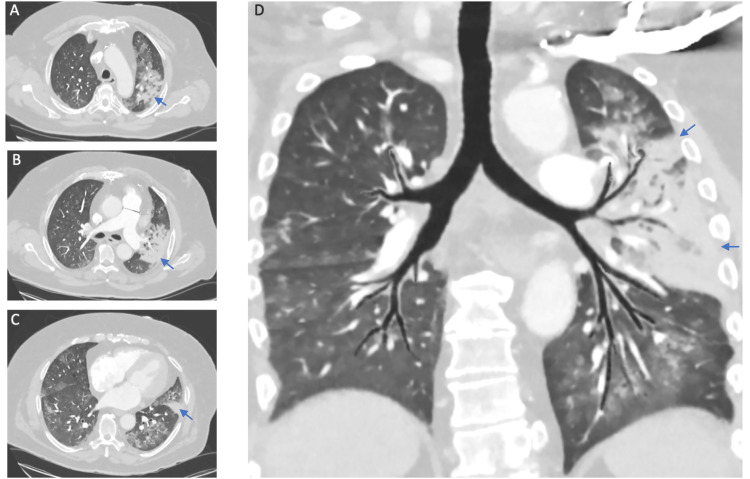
Chest computed tomography angiography Confluent area of consolidation with an air bronchogram in the left upper lobe and diffuse ground glass opacities. A-C: axial views in the upper, medium, and lower lung zones; D: coronal view
